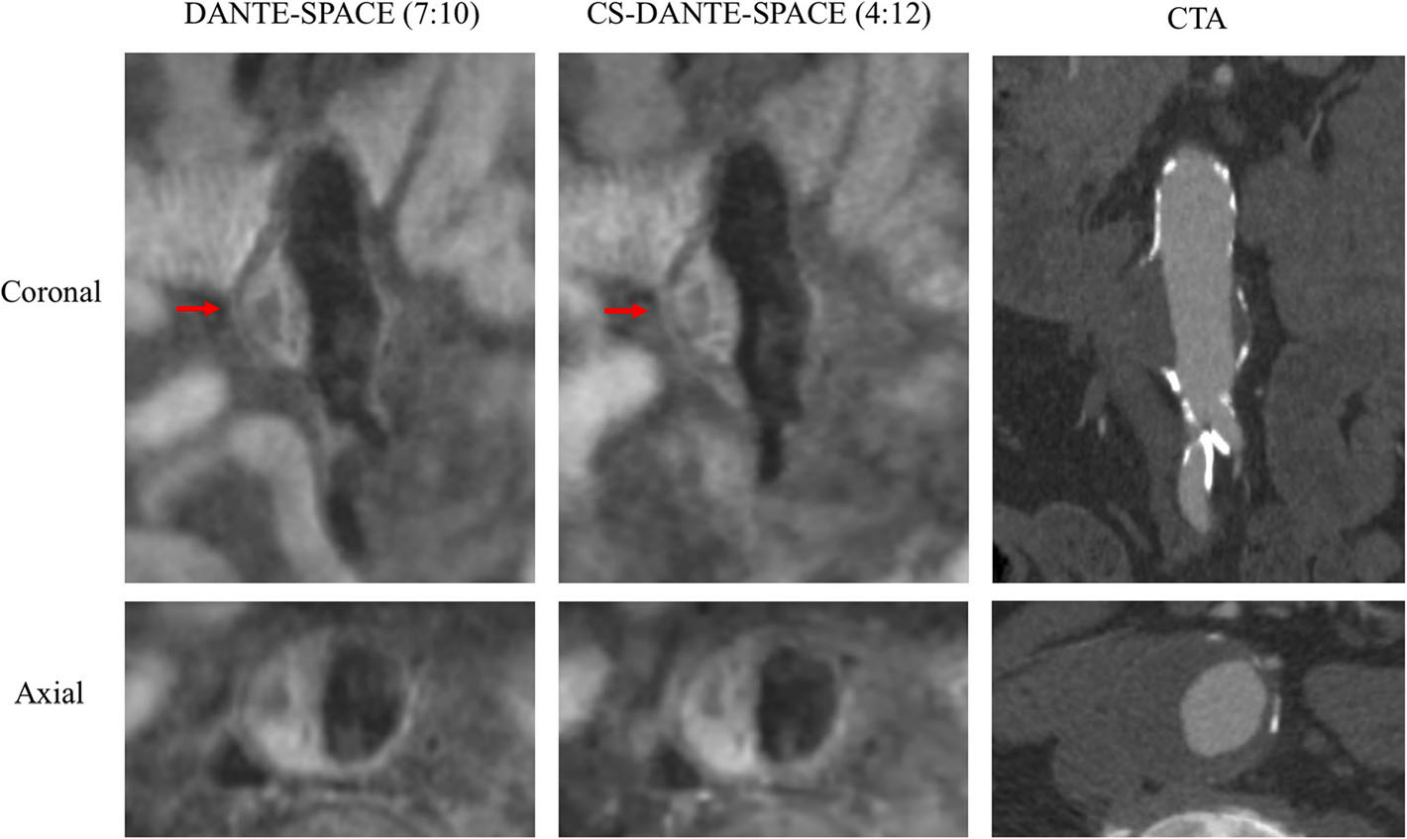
A patient with a 4.7 cm aneurysm. CS-DANTE-SPACE achieved comparable image quality with DANTE-SPACE, but with a 41% scan time reduction. Reference CTA 2 month before CMR is shown on the right. Red arrows show the level of maximal diameter. Heterogeneous intraluminal thrombus (ILT) was demonstrated in both sequences. A sharper image was noted for CS-DANTE-SPACE, possibly due to the reduced motion artifacts because of shorter scan time
